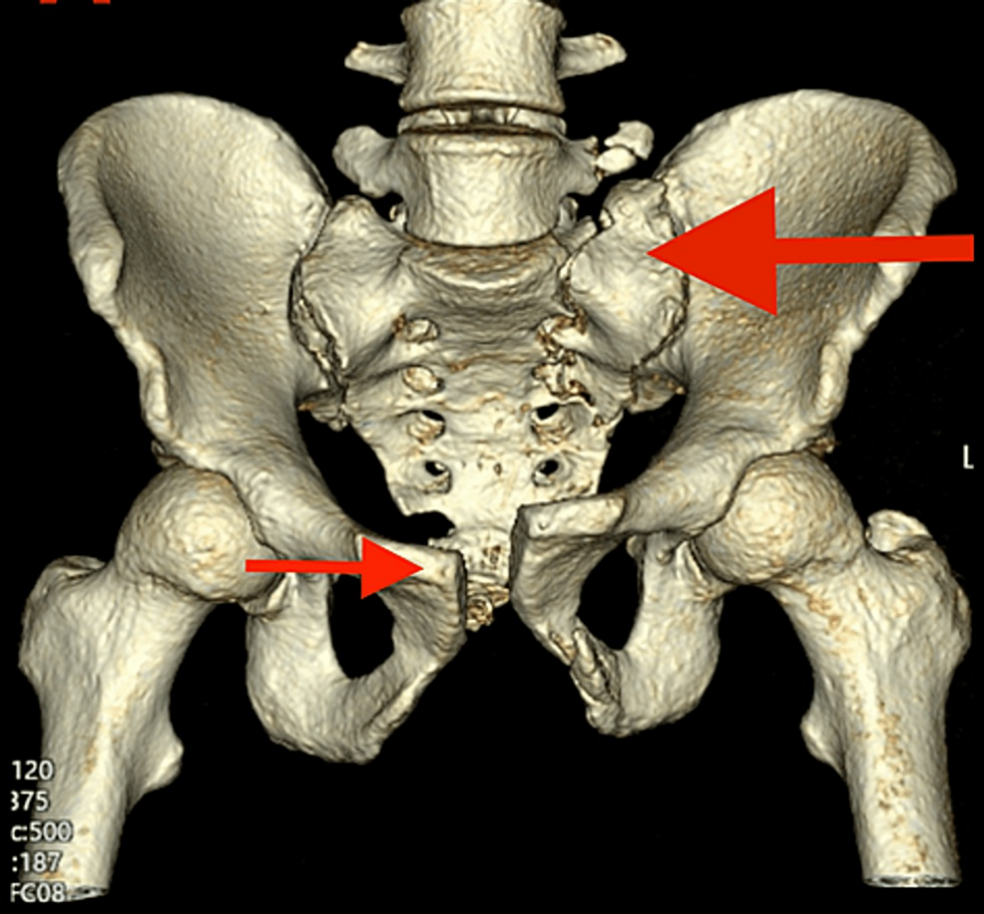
CT scan of the first patient showing symphysis pubis diastasis with left sacral ala fracture.
